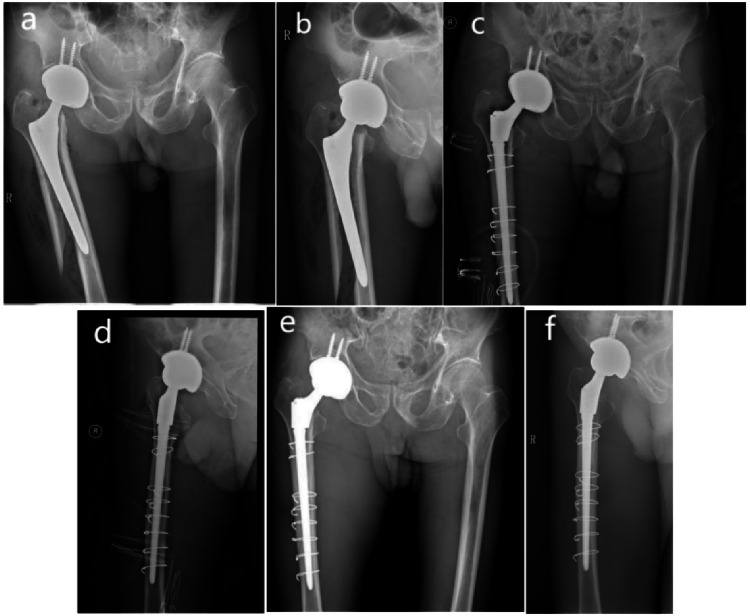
Radiographic examination of a Vancouver B2 PFFs of the right femur in a 78-year-old male (a, b) treated by simple femur revision with modular femoral stem and wires. The postoperative radiograph showed an anatomic reduction (c, d). The radiograthic examination taken at follow-up, 6 years later, showed a fracture healing without deformity or shortening (excellent result,according to Beals and Tower’s criteria) (e, f). At clinical evaluation, the HHS scored 90 points
